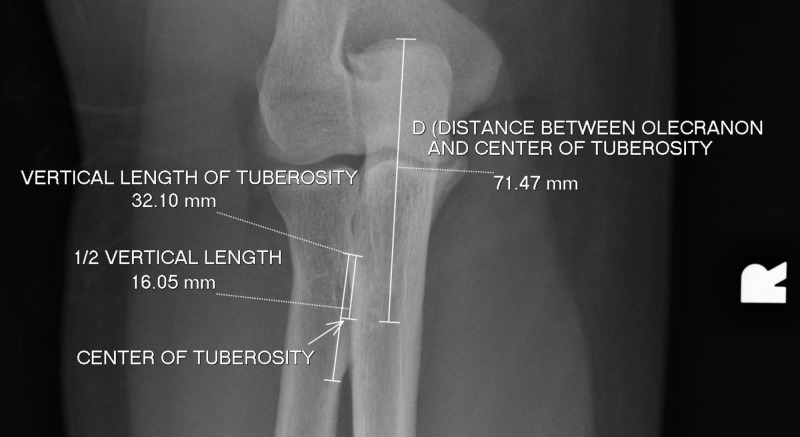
Measurement of olecranon to tuberosity distance. Radiographic measurement of the distance in mm between the olecranon tip and the center of the radial tuberosity using anteroposterior (AP) plain film X-rays.
